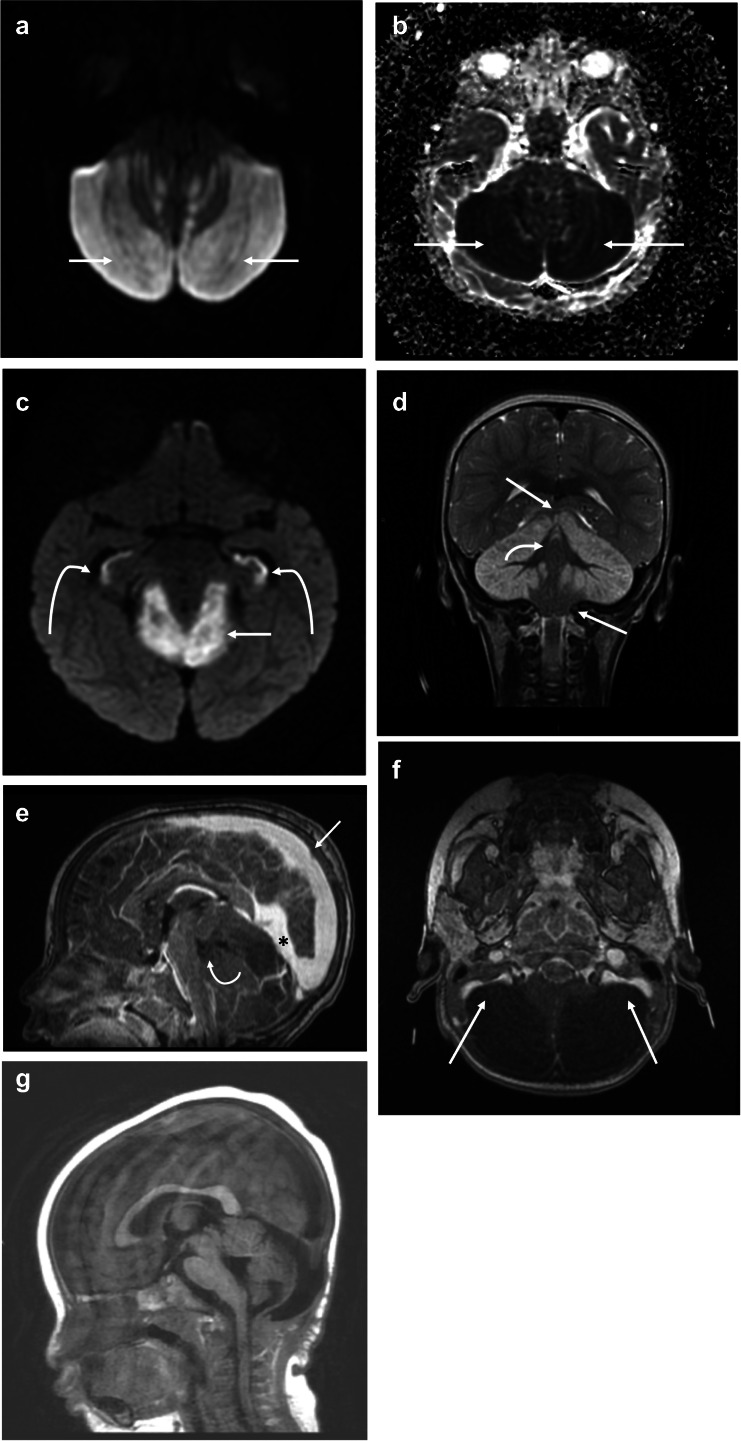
a Axial diffusion-weighted image (DWI) b1,000 s/mm^2^ showing diffuse cerebellar restricted diffusion confirmed as profound decreased signal on ADC map (b). c Axial DWI shows symmetric hippocampal restricted diffusion (curved arrows). Superior transtentorial herniation with cerebellar cortical restricted diffusion (straight arrow). d T2-weighted coronal image shows diffuse cortical cerebellar hemispheric edema with superior transtentorial herniation and bilateral downward cerebellar tonsillar herniation (straight arrows). Notice relative sparing of edema in cerebellar vermis (curved arrow). e Sagittal contrast T1 midline image demonstrating severe brainstem compression. Notice severely dilated sagittal (arrow) and straight (*) dural sinuses and compression of fourth ventricle (curved arrow). f Axial contrast T1 image at the level of the medulla confirming symmetric bilateral sigmoid sinus compression (arrows). g Follow-up T2 axial image obtained 2 months after initial presentation shows bilateral cerebellar encephalomalacia
